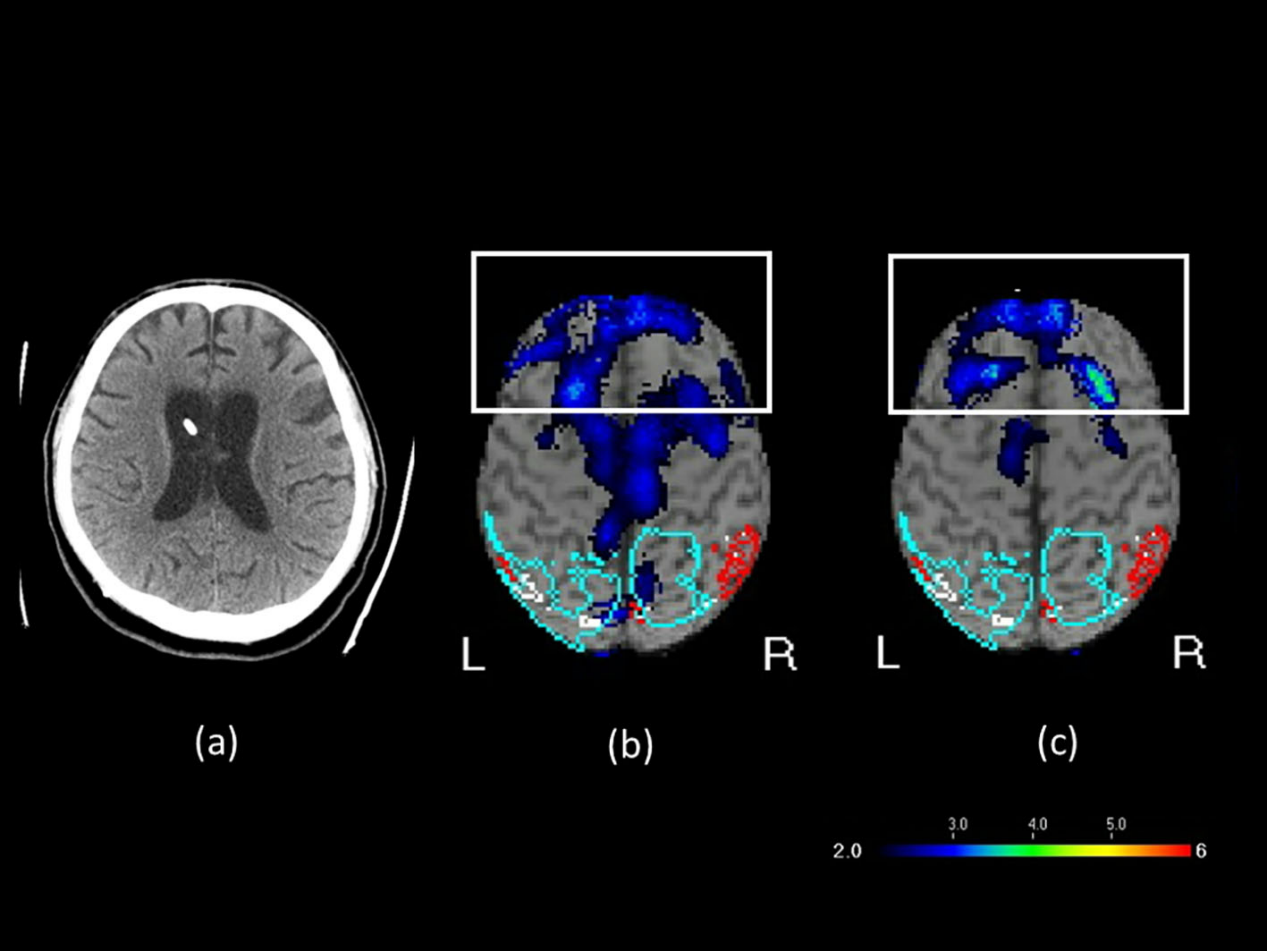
(a) Brain CT image before rTMS treatment, (b) SPECT brain image before rTMS treatment, and (c) SPECT brain image after rTMS treatment in Patient A. In the transverse SPECT view, the white rectangular box highlights the frontal lobe. The color scale represents varying levels of blood flow or functional activity, with cooler colors such as blue and purple indicating regions of hypoperfusion.
